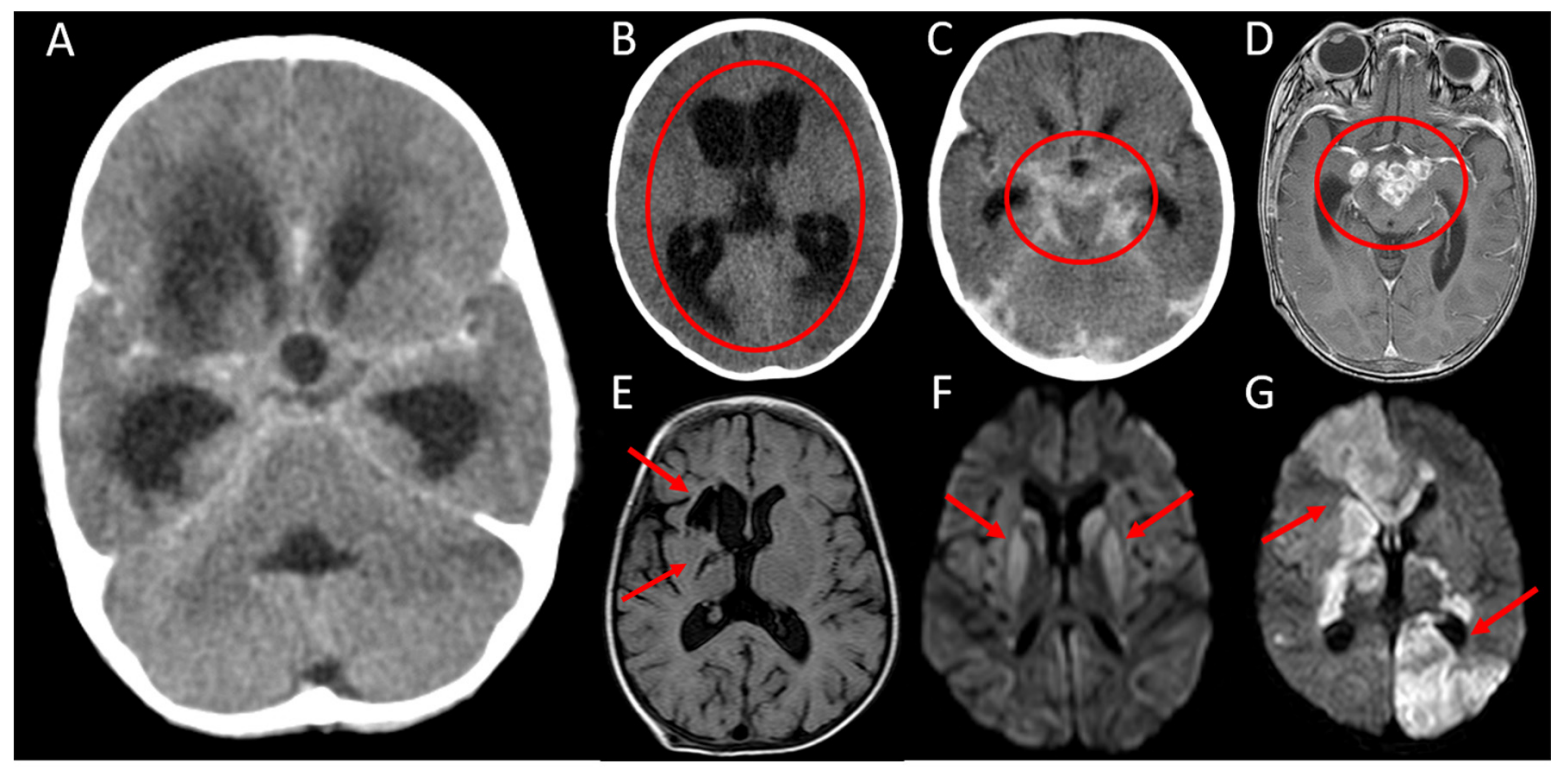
Neuroimaging findings. A: Axial computed tomography (CT) with contrast, demonstrating the neuroimaging triad consistent with tuberculous meningitis (TBM) - hydrocephalus, basal meningeal enhancement and a right basal ganglia infarct;
